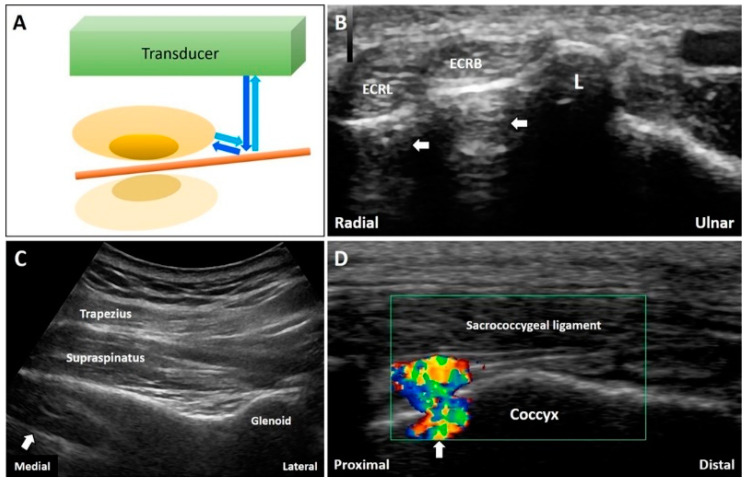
When the sound waves encounter a reflective interface, the reflected beam would cast a mirror image at the opposite side of the interface (A). The mirror artifacts can be seen at many body regions like the forearm (B), supraspinatus fossa (C), and coccyx (D). White arrow: mirror artifact. ECRL: extensor carpi radialis longus tendon; ECRB: extensor carpi radialis brevis tendon; L: Lister’s tubercle Blue arrows: the projected and reflected US beams. Brown pillar: the reflective interface. Green box: the color box for detection of Doppler signals.
